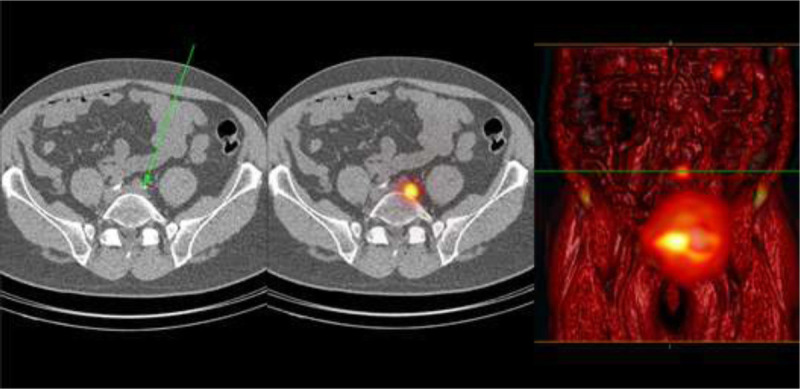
A blurry image is seen without an obvious focus or enlarged lymph node on SPECT/CT images, shine-through phenomena with nonvisualization of the SN, where the injection site shines through and could outshine possible SN nearby. This could lead to false-negative findings.
